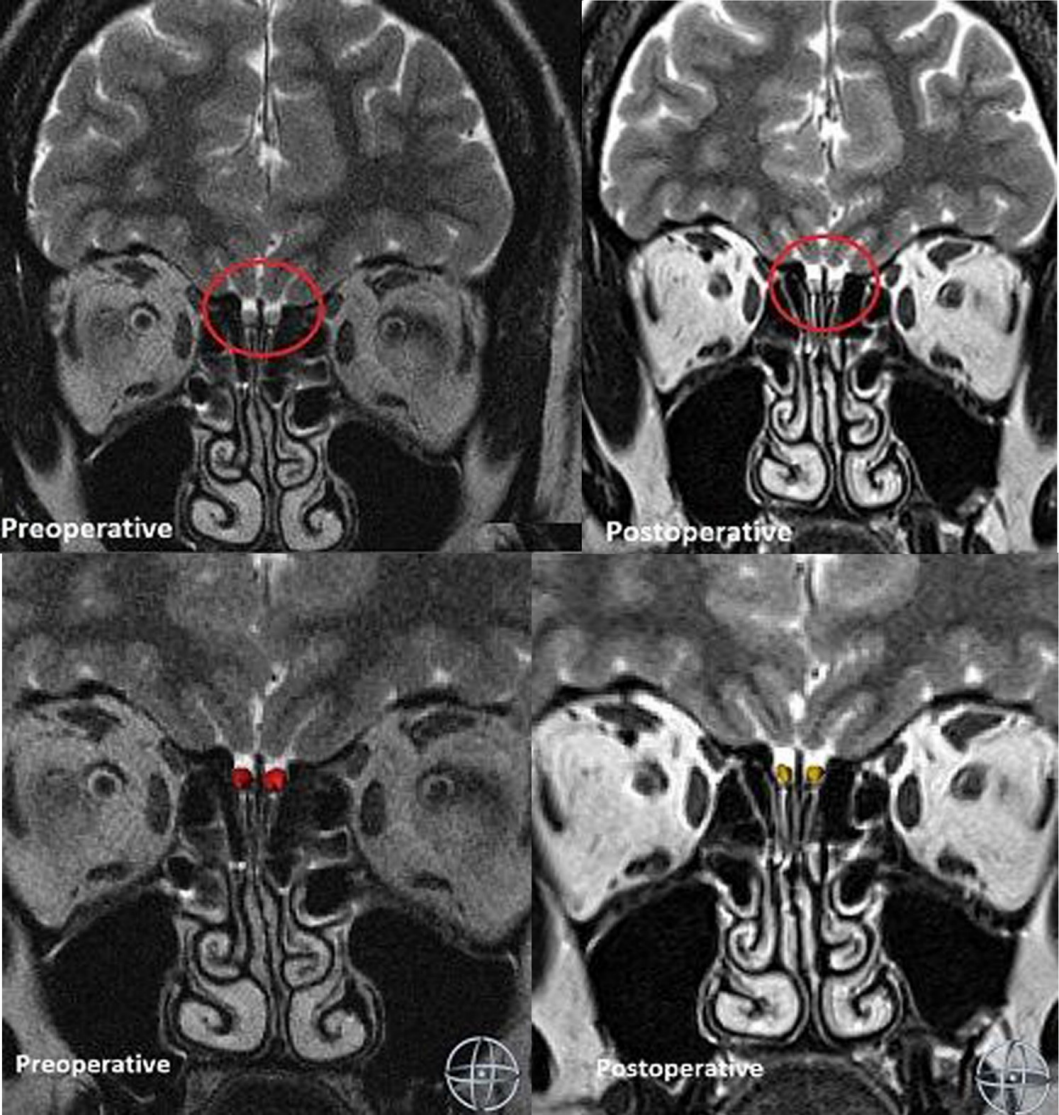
Segmented OB in a 60 years old female patient with pituicytoma pre- and postoperatively on the basis of T2 coronar images. Postoperative volumetry was performed 13 months after transsphenoidal tumor enucleation. The protocol included T2-weighted 2D turbo spin echo (TSE) sequence in the coronal plane (matrix 256×256; field of view 120×120; slice thickness 2.0 mm; number of slices 30). Olfactory bulbs were smaller postoperatively.
